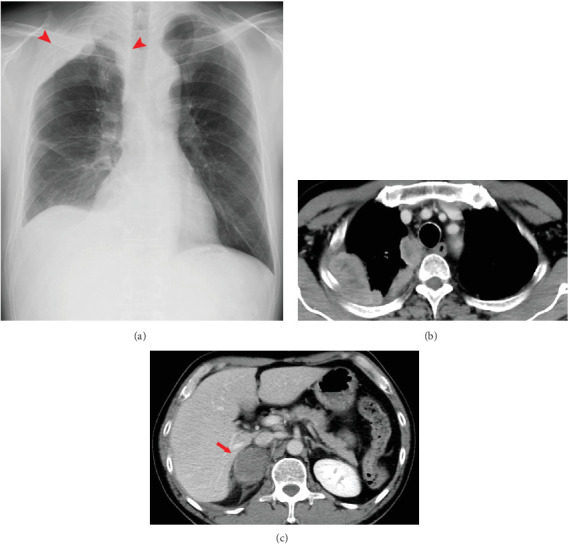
(a) Chest x-ray revealing right apical pleural thickening. (b) CT image demonstrating right continuous pleural thickening (arrowheads), including the mediastinal pleura and lymph nodes, (c) with a concurrent presence of right adrenal gland tumor (arrow). CT, computed tomography.
